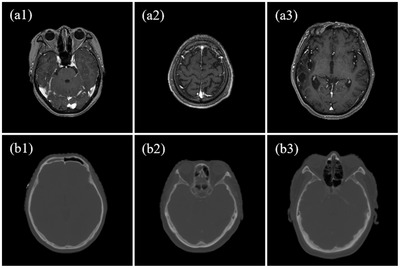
Unpaired training data. (a1–a3) are randomly selected MRI images in the training set, and (b1–b3) are the corresponding CT images. We trained all models in this work using MRI and CT images of different patients at different anatomical locations.
